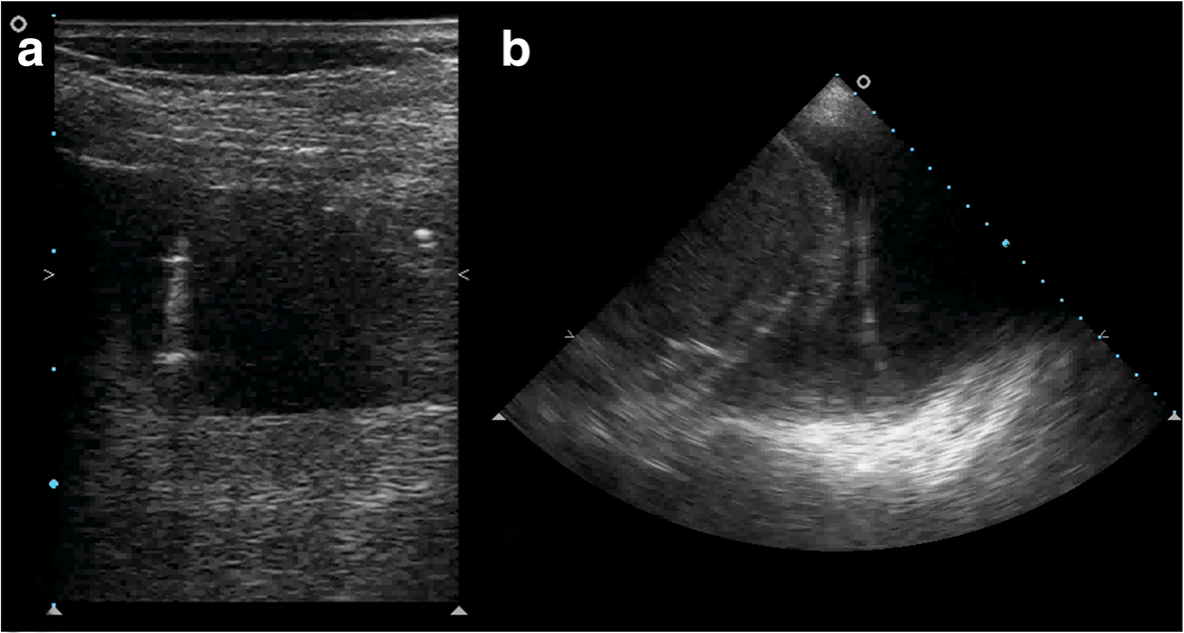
Right pleural effusion seen through a linear (a) and convex (b) ultrasound probe. The hyperechoic line visible within the pleural hypoechoic effusion is the metallic wire inserted to guide the introduction of the pleural drainage
